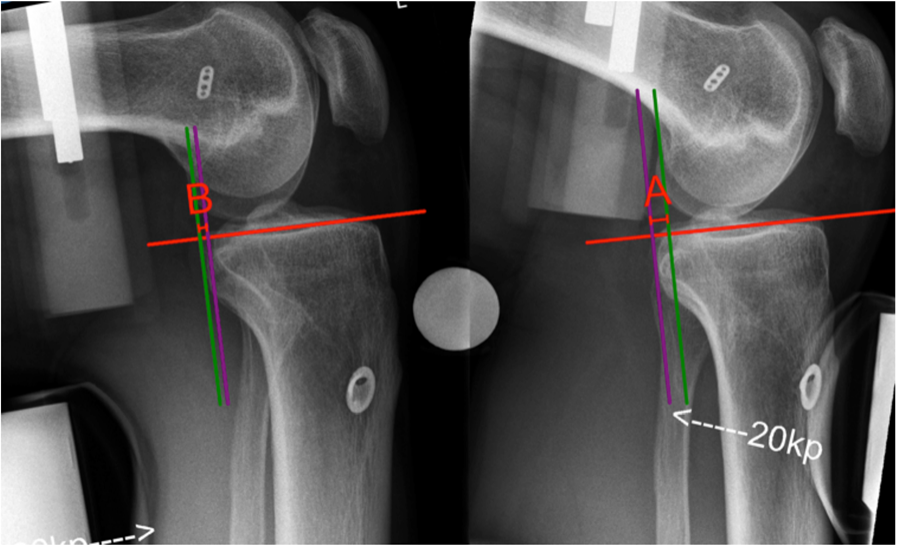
Posterior tibial displacement (A) and anterior tibial displacement (B) on stress radiograph with an applied load of 20 kp.
